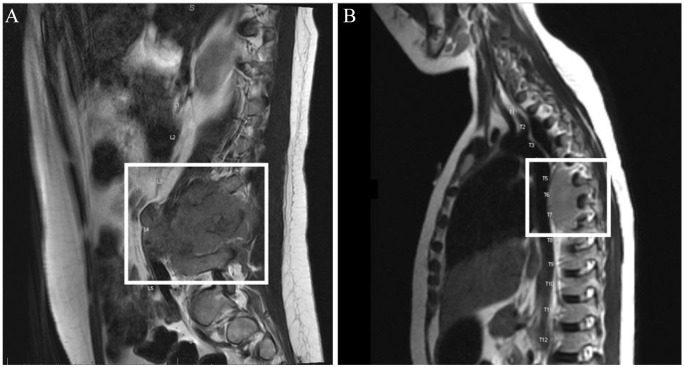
(A) Sagittal pre-contrast T1-weighted fat-saturated magnetic resonance imaging of lumbar spine demonstrates marrow replacing lesion within the L4 vertebral body with extension into the epidural space and associated cortical destruction of the anterior and posterior cortex with bulging of the anterior and posterior longitudinal ligament and (B) epidural extension of tumor extends along the dorsal aspect of the vertebral bodies from the L3 to the L5 level. There is circumferential epidural tumor which extends from the L3-L4 level to the L5 level. There is involvement of the spinous processes as well as the bilateral pedicles.
